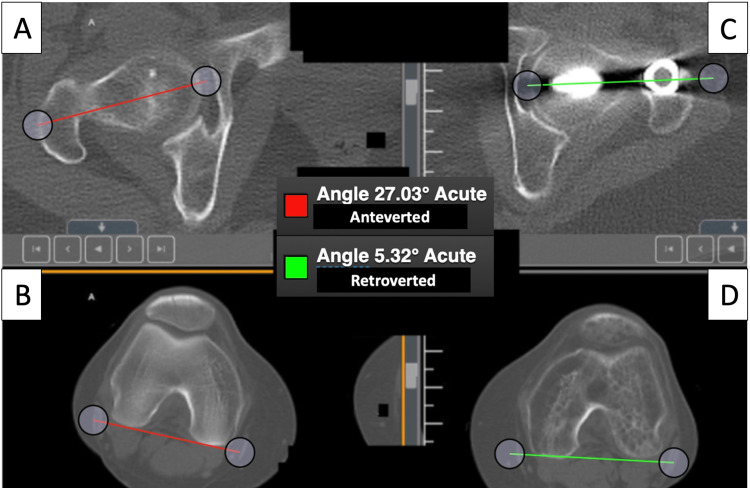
Pre-correction CT scanogram with pre-correction femoral rotation measurement obtained for the left and right extremity with the Bonesetter application. The contralateral femur (A, B) measures 27 degrees of anteversion (red lines). The operative femur (C, D) with the presence of an IM nail measured 5 degrees of retroversion (green lines). A 32-degree difference is outside the acceptable range of <15 degrees compared to the contralateral side. ° = degree
